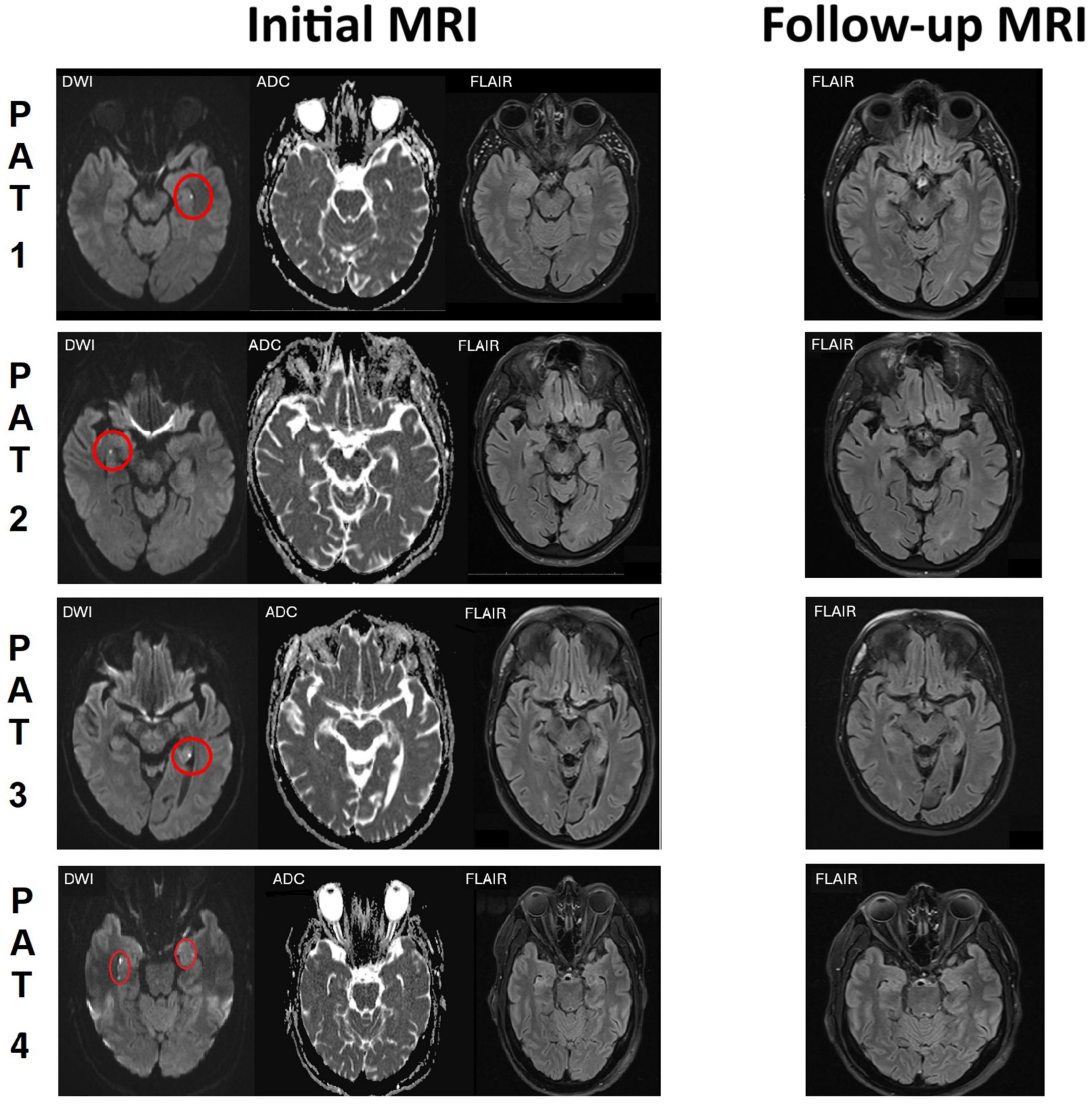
Initial MRI and follow-up MRI of the patients. Left column (initial imaging): DWI on the left, ADC-map in the middle, FLAIR-weighted image on the right. Punctate hippocampal DWI lesions in all 4 patients circled in red. Right column (follow-up imaging after 8 days - 3.5 months): FLAIR-weighted imaging.
